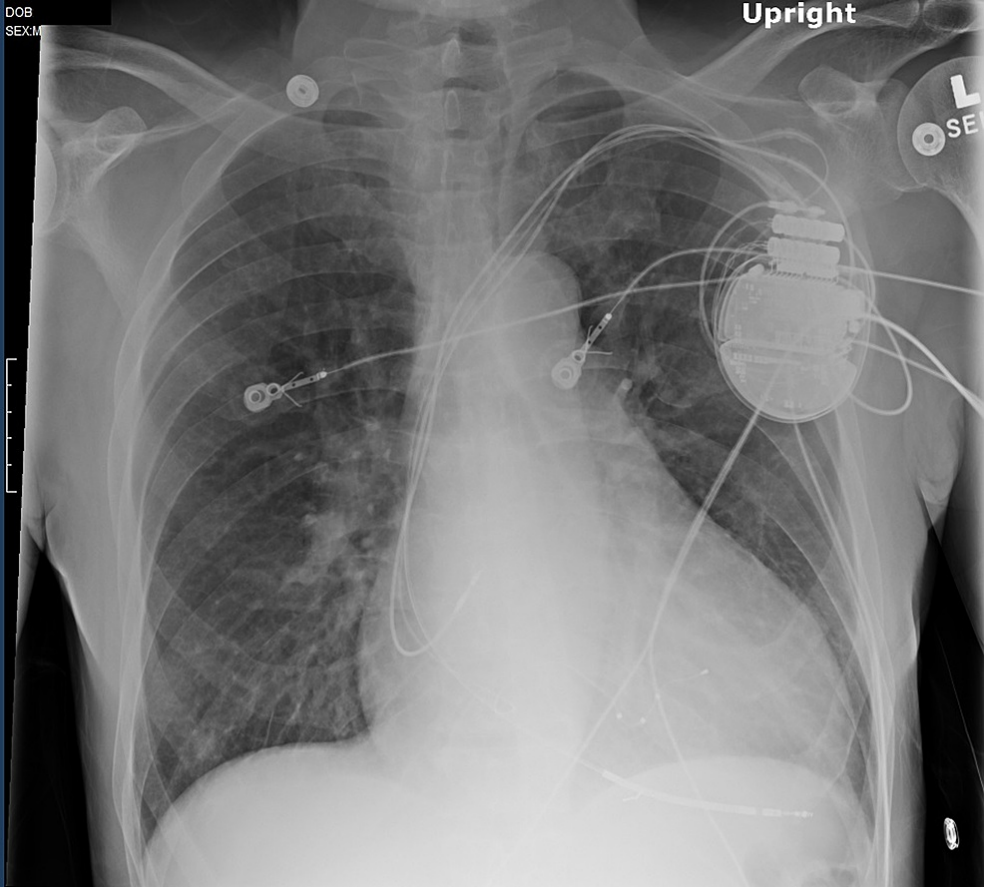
Post-procedure chest X-ray. A portable anteroposterior chest x-ray was obtained after the procedure. An implantable cardioverter-defibrillator pace/sense/defibrillator lead was placed in the right ventricle septal apex where appropriate function was demonstrated. The other leads can be seen at the right atrium and the coronary venous sinus. The leads were sutured to the pectoralis muscle with a suture sleeve. Adequate hemostasis was ensured and the pocket was flushed with an antibiotic solution. The leads were connected to the generator and the system was placed into the pre-pectoral pocket. The wound was closed with layers of absorbable suture. The procedure was tolerated well and there were no complications.
